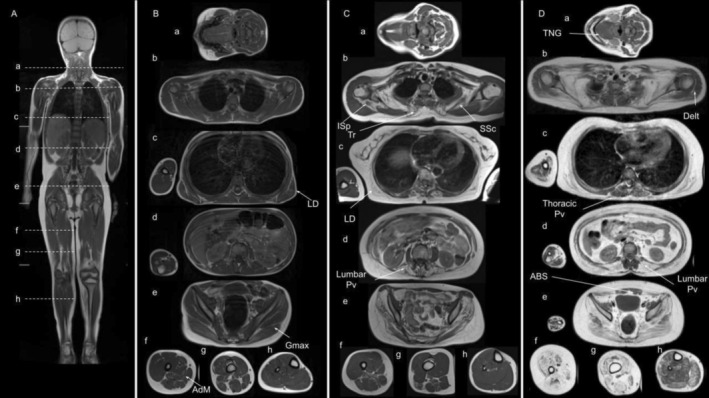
Progressive muscle involvement in sarcoglycanopathies. (A) Localizer. Coronal view of whole‐body MRI, displaying the corresponding axial slices (a–h). (B) P24, LGMDR5, mildly affected: Initial involvement of latissimus dorsi (LD), gluteus maximus (Gmax), and adductor magnus (AdM). (C) P35, LGMDR3, intermediate stage: Advanced involvement of subscapularis (SSc), infraspinatus (Isp), and trapezius (Tr) and complete replacement by fat of LD. Lumbar paravertebral muscles (Lumbar Pv) are also severely affected. (D) P1, LGMDR3, severe stage (able to walk only with support): Severe and diffuse involvement of lower paravertebral, pelvis, and thigh muscles with complete sparing of the tongue (TNG) and relative preservation of thoracic paravertebral muscles (Thoracic Pv); deltoid (Delt) and proximal arm muscles are mildly affected. Rectus abdominis (ABS) is also preserved in contrast to obliquus and transversus abdominis.
